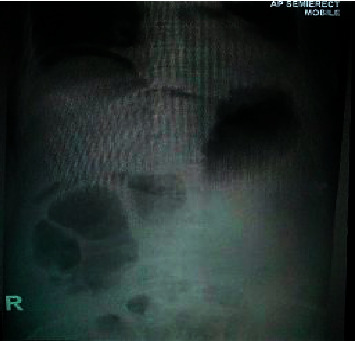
Plain X-ray abdomen; gas under diaphragm positive, gas-filled distended stomach, and some bowel loops.
